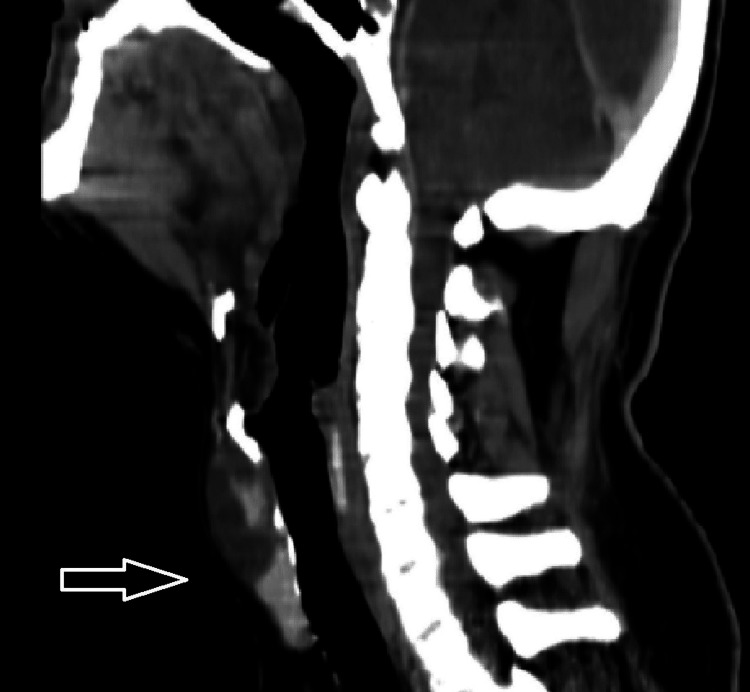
Preoperative CT scan image showing a well-defined midline infra-hyoid multicystic lesion, with slight capsular enhancement, compatible with a thyroglossal duct cyst (white arrow).
